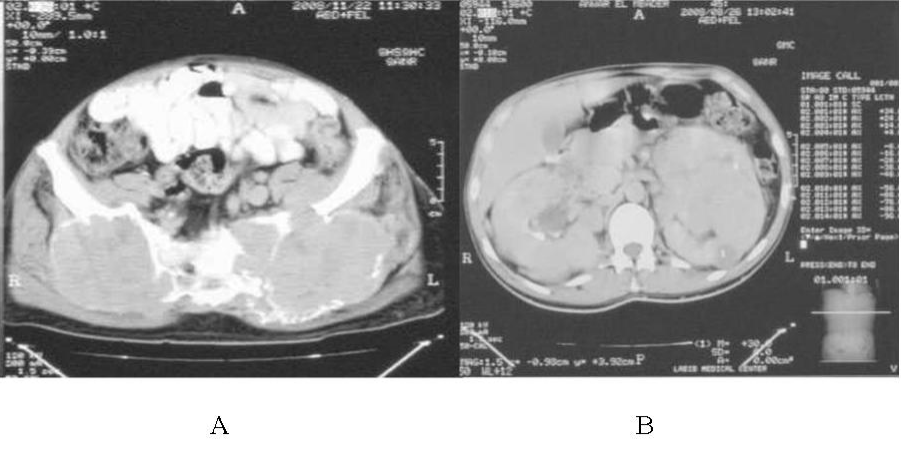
(A) Axial MRI images showing bilateral sacroiliac heterogeneously enhancing masses. (B) Abdominal CT scan showing large bilateral renal metastases.
